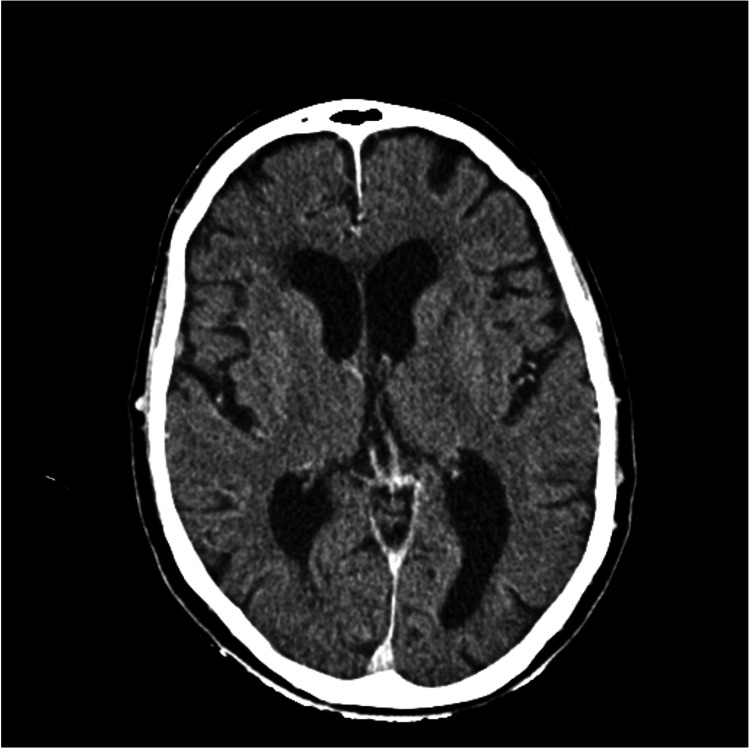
Computed tomography angiography of the cerebral vessels after the first event.
